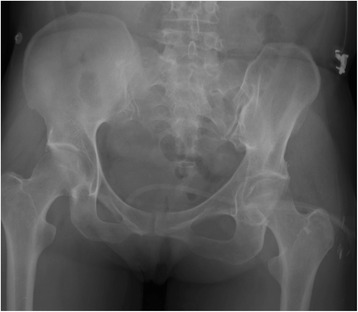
Pre-operative anteroposterior radiograph of pelvis demonstrating lack of evidence of left femoral neck fracture
